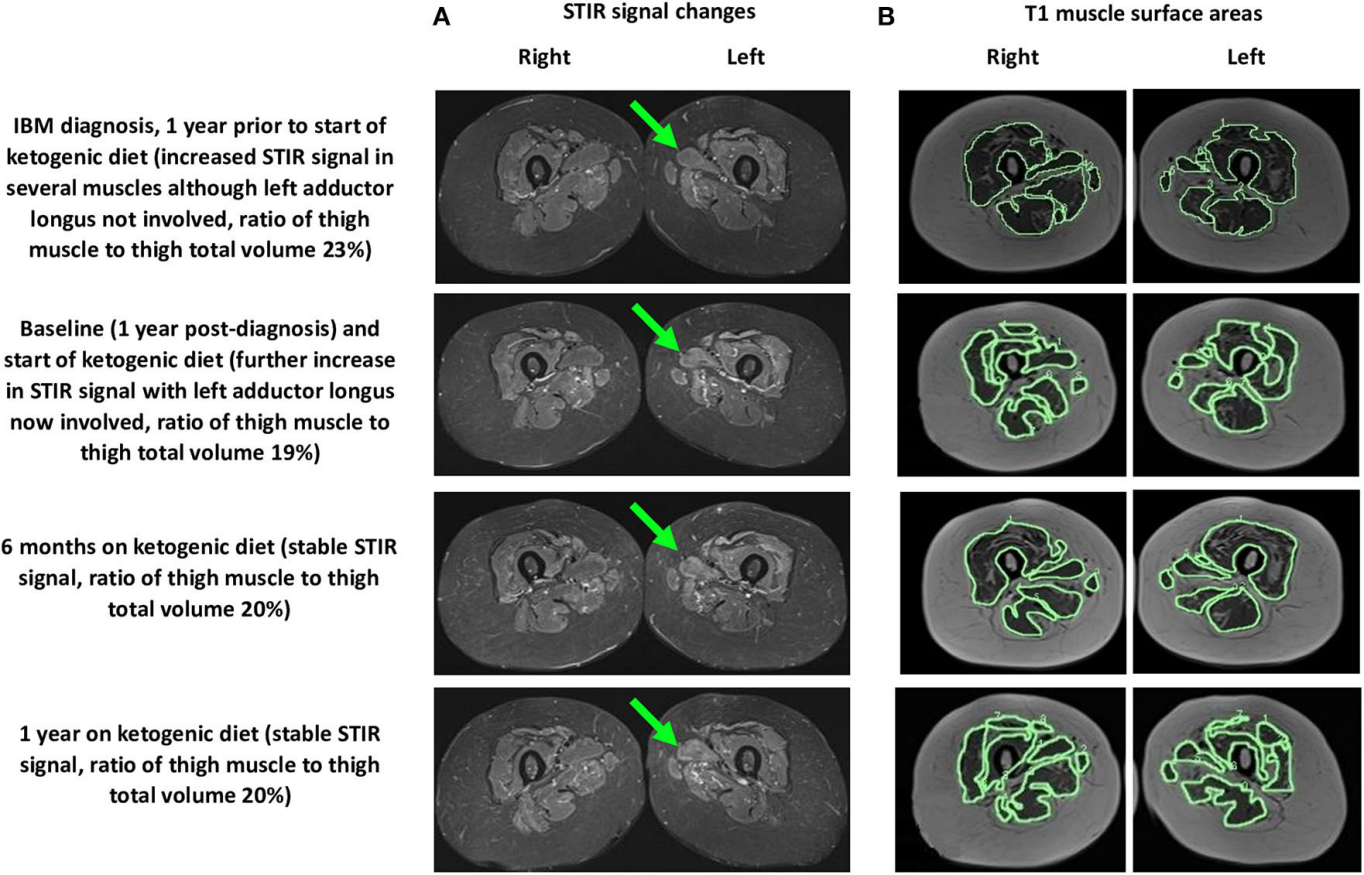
MRI (axial views) of the bilateral thighs showing thigh muscle (A) STIR signal changes (left adductor longus shown by green arrow) and (B) T1 muscle surface areas (outlined in green, only axial plane 3 of 5 is shown) at 1 year prior to starting the ketogenic diet and at baseline, 6 months, and 1 year after starting the diet.
